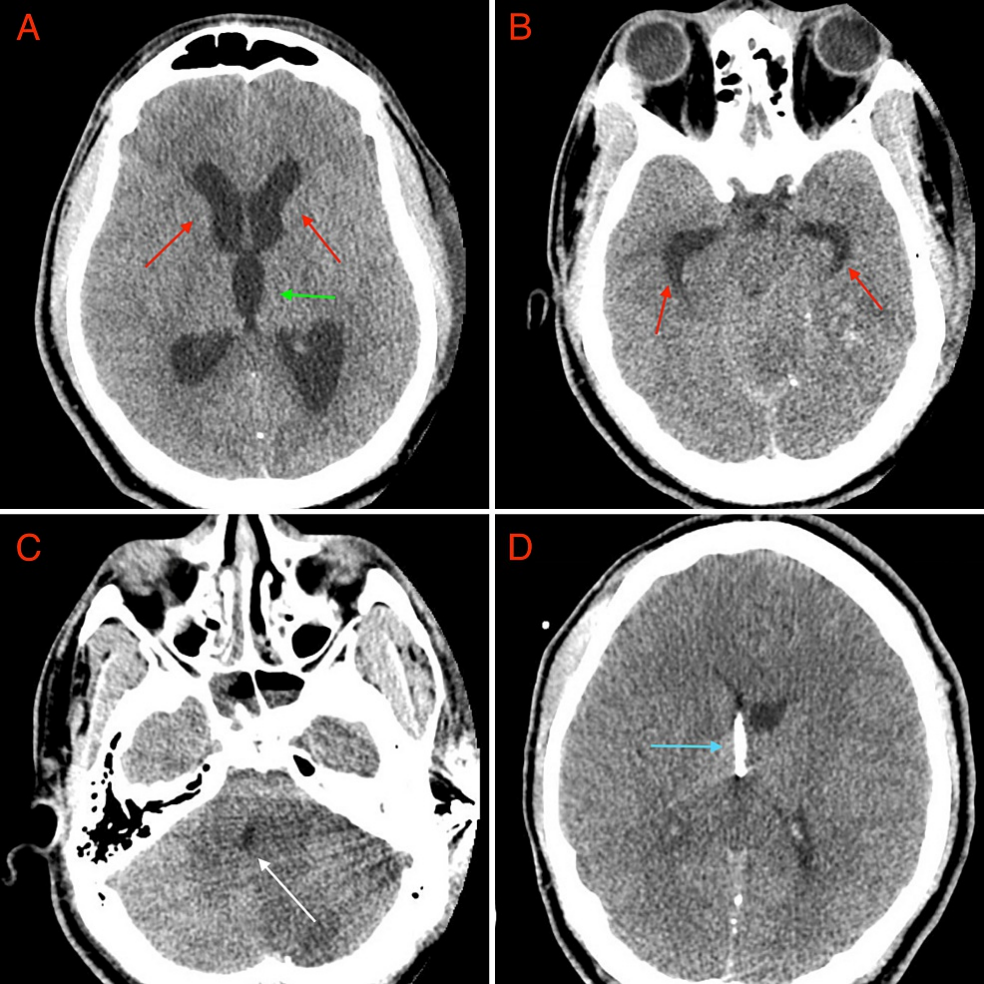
Repeated diagnostic imaging showing cerebellar edema, obstructive hydroecephalus, and external ventricular drain (A-D) 2A-B: Axial CT images showing ventriculomegaly of the lateral (red arrows) and third (green arrow) ventricles secondary to obstructive hydrocephalus; 2C: Axial CT image showing cerebral edema, mass effect, and compression of the fourth ventricle (white arrow); 2D: Axial CT image showing postoperative placement of external ventricular drain (EVD) (blue arrow).
